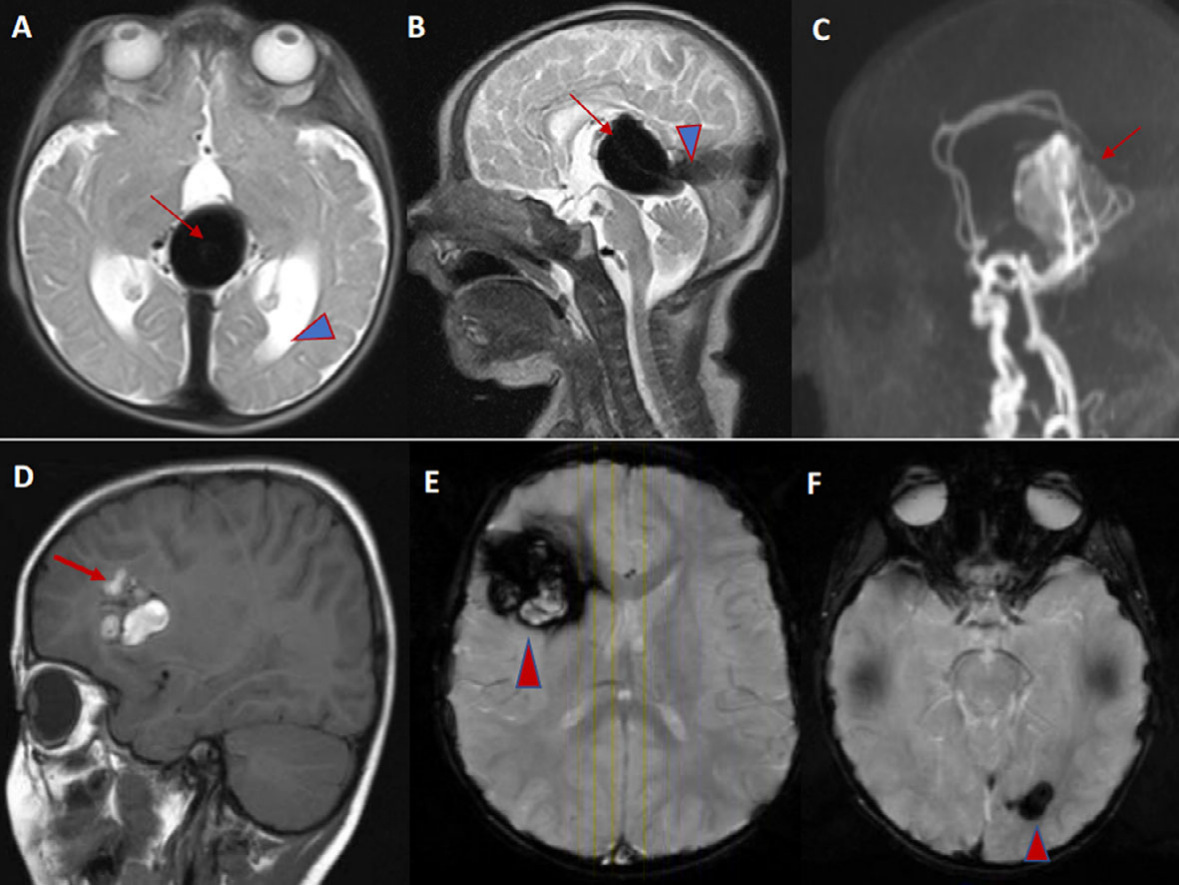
A 6‐day‐old boy with high output heart failure, pulmonary hypertension, and reversed diastolic aortic flow. Axial & Sagittal T2W MRI brain showing dilated medial prosencephalic vein (arrow) and persistent falcine sinus (arrowhead) (A, B). The visualized brain parenchyma is normal for age. Sagittal 3D reconstruction of noncontrast MRA head demonstrating arterial pedicles from the limbic arcade (arrows) supplying the vein of Galen malformation (C). Patient with familial Cavernoma. Axial GRE (blood‐sensitive) images demonstrating the classical “popcorn” appearance of a large right frontal lobe CM (arrow) with classical imaging findings of blood in different stages of breakdown (both high & low T2 signal, high and low T1 signal), hypointense rim on T2W images and surrounding vasogenic edema (D, E). Multiple foci of susceptibility in the right and left hemispheres of the brain (arrowheads) compatible with multiple cavernous malformations (F).
